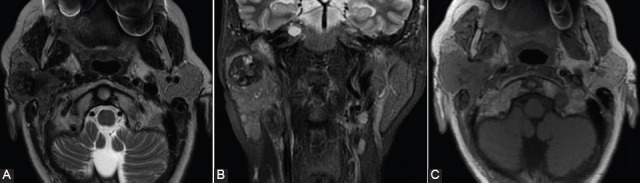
Conventional magnetic resonance imaging appearance in a 70-year-old patient with a malignant tumor of the right parotid gland (carcinoma ex pleomorphic adenoma). (A) T2-weighted image (WI) sequence, (B) short tau inversion recover [STIR], and (C) non-enhanced T1-WI sequences showing an inhomogeneous lesion in the right parotid gland, with infiltrative contour.
